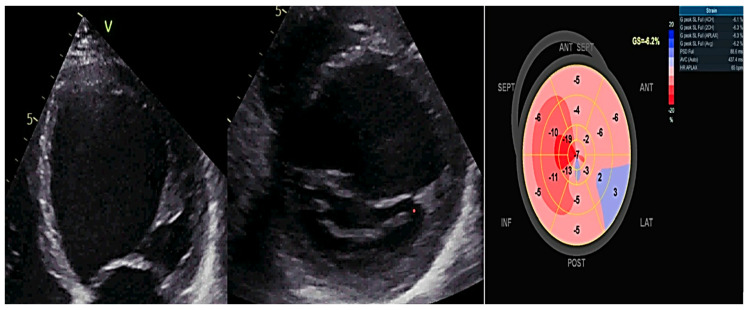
Familial dilated cardiomyopathy. Two-dimensional trans-thoracic conventional echography in apical longitudinal and parasternal short axis vi ews. Bull’s eye myocardial deformation with diffuse reduced left ventricular longitudinal peak strain patterns.
